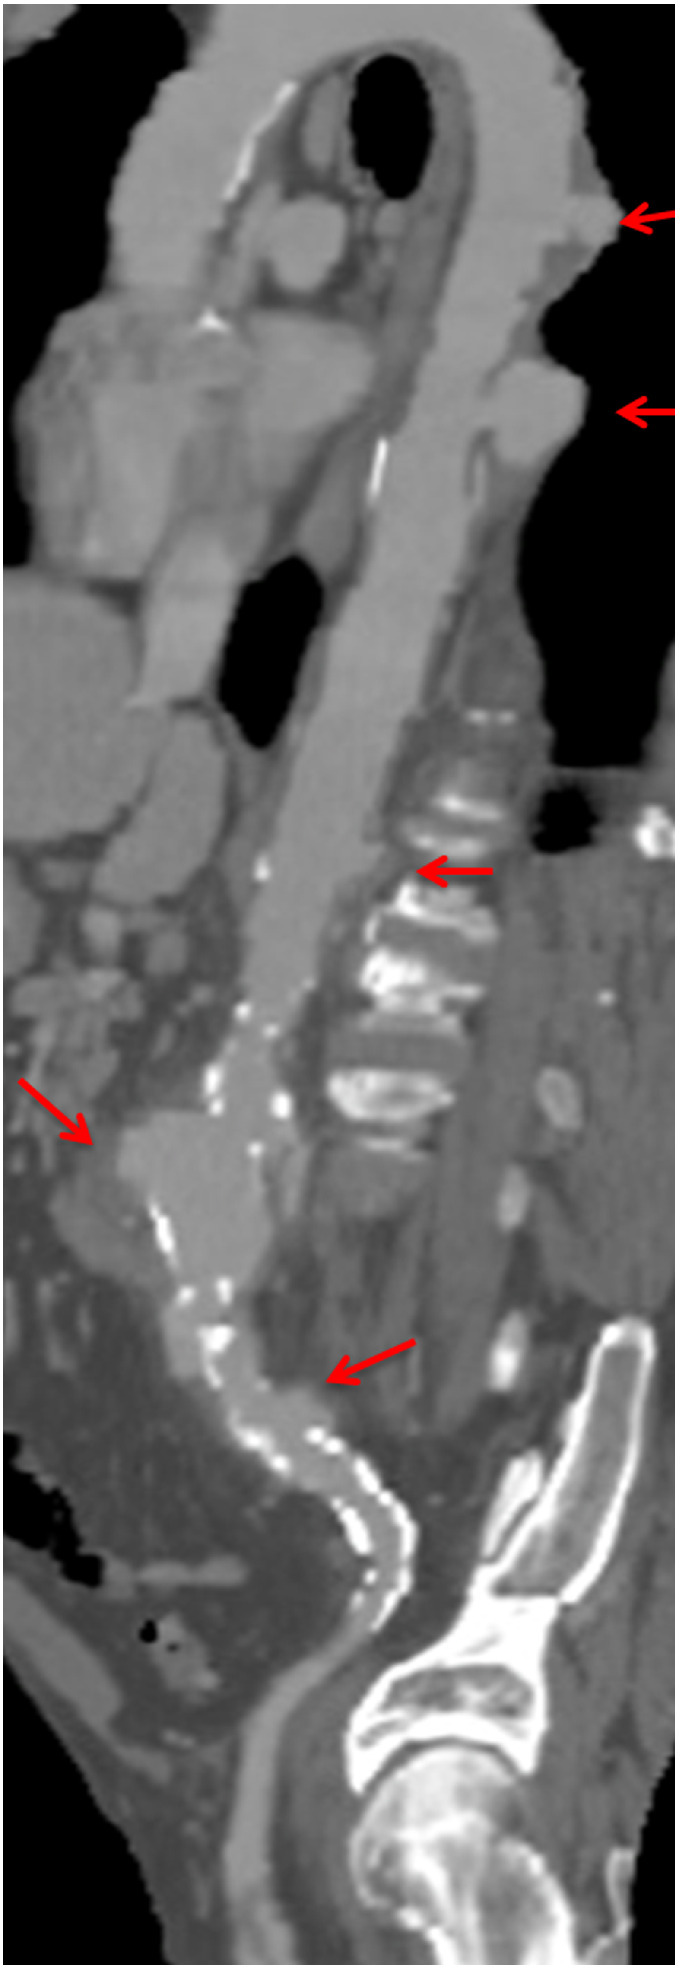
Fig. 1 Axial computed tomography images showing thoracic penetrating aortic ulcers, abdominal and left common iliac artery aneurysms, with chronic occlusion of the right iliac axis.
